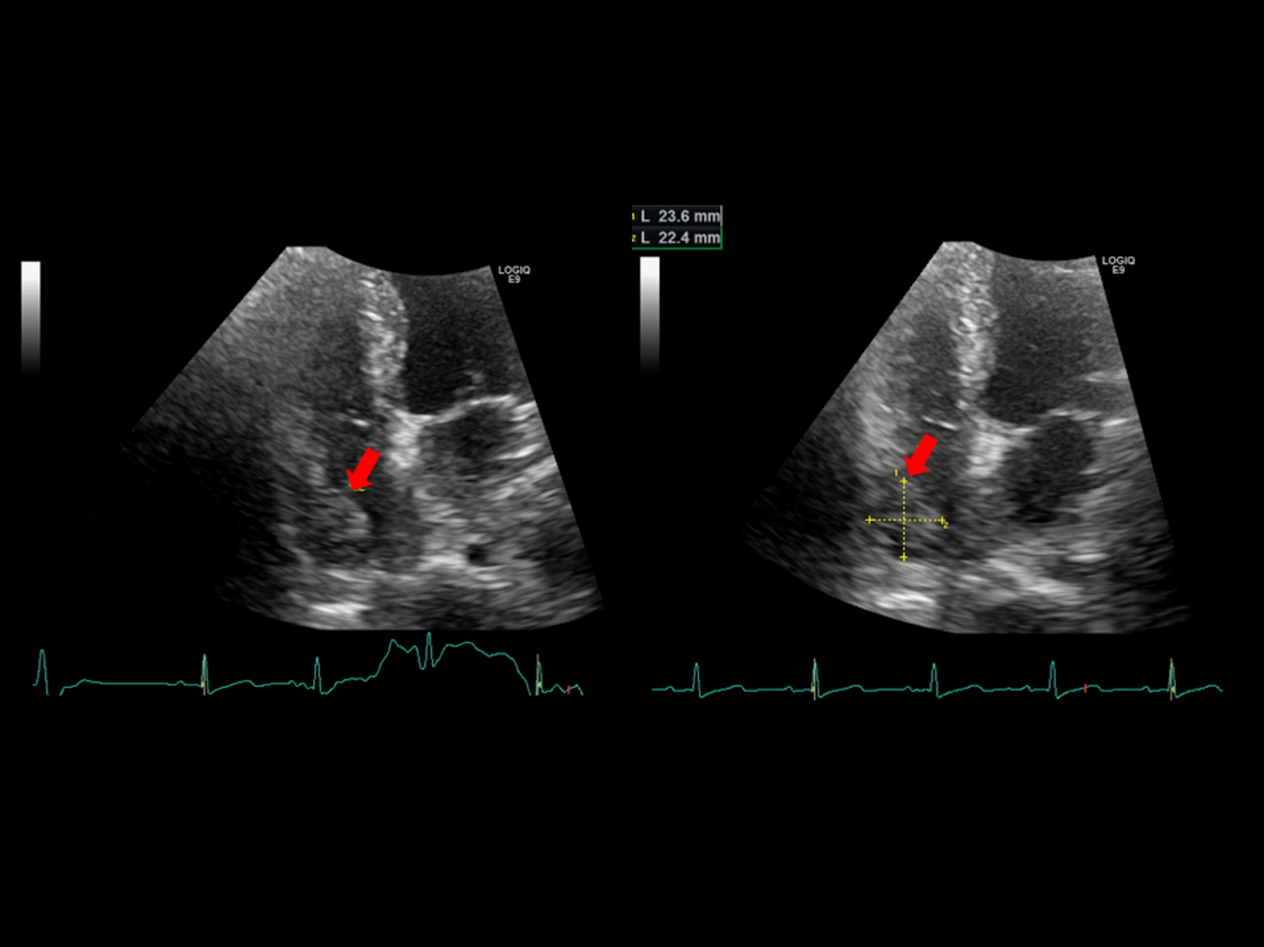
Cardiac ultrasound showing tumor. Four-chamber view from the apex. Red arrow denotes IVC tumor protruding into the right atrium (23.6 x 22.4 mm in size). IVC, inferior vena cava
